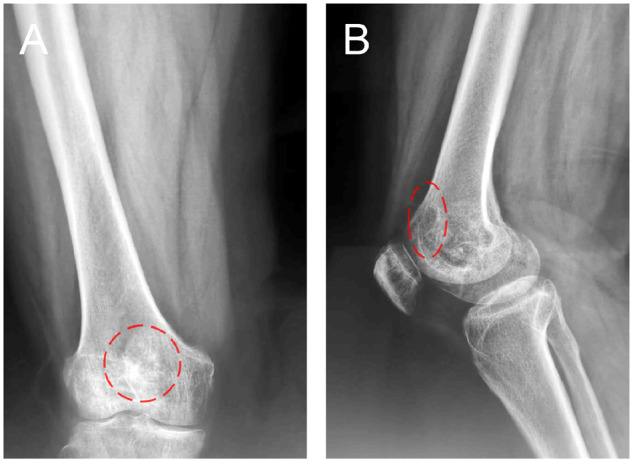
Frontal (A) and lateral (B) views of plain radiography at 6 months after the operation (the surgical site is noted by dashed circle in each picture).
